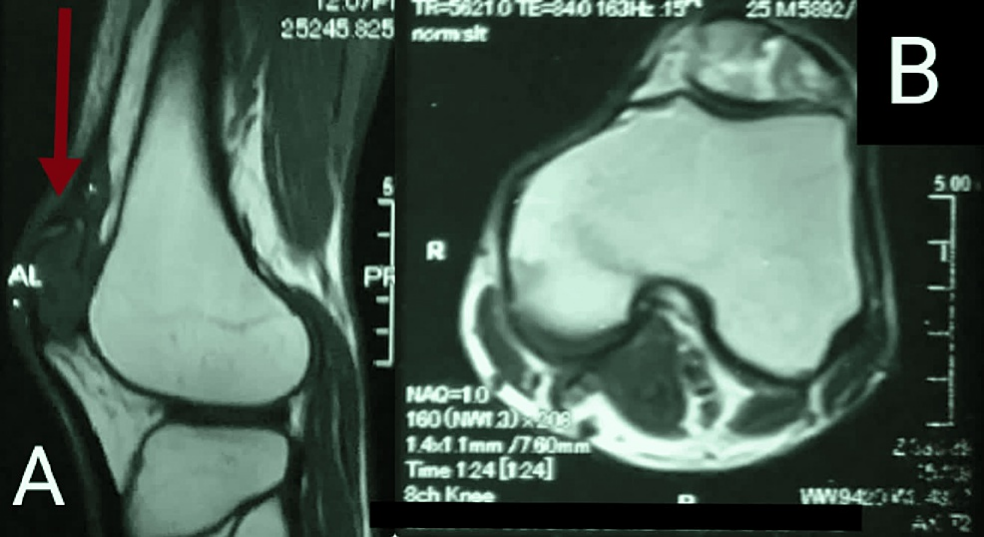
Sagittal (A) and axial (B) MRI slices representing the details of the confined heterogeneous textured patellar lesion. Involvement of the proximal femur and tibia appears negative.
